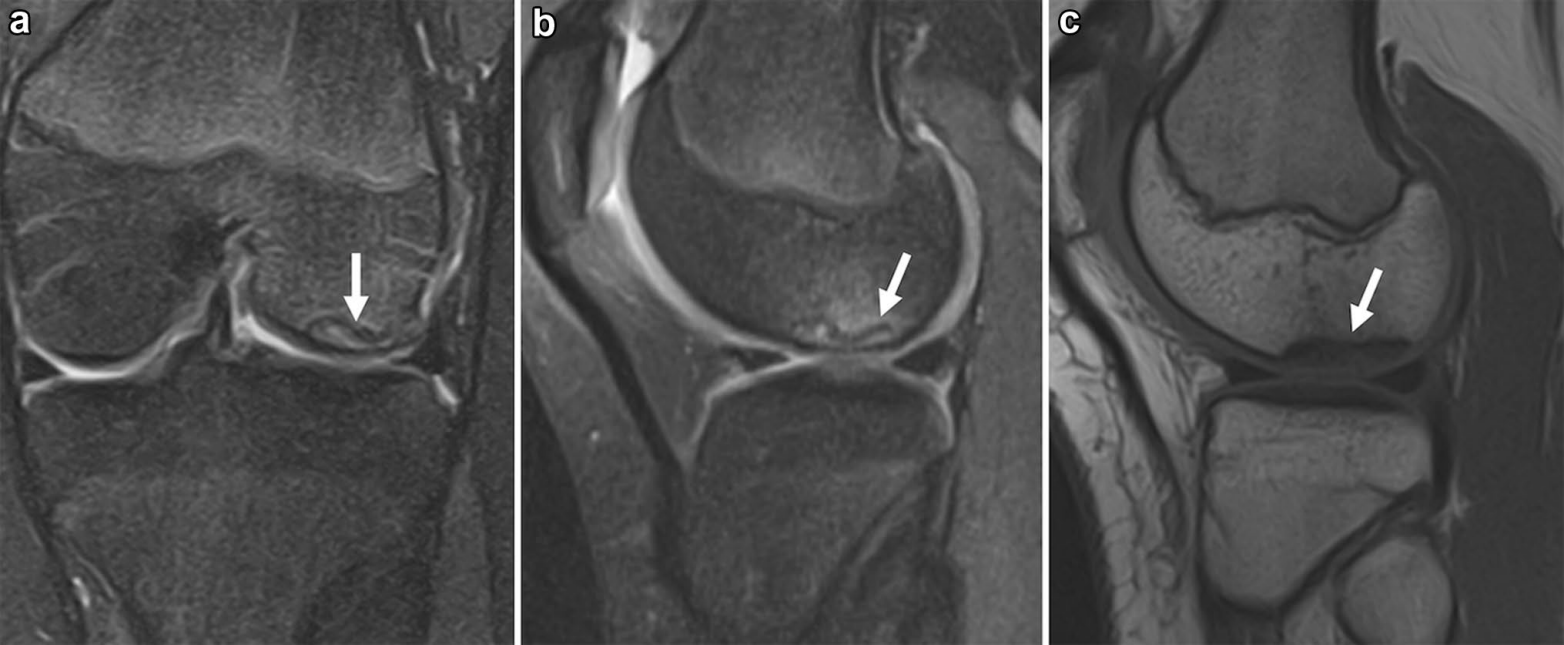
a Coronal FS-PDWI, b Sagittal FS-PDWI, and c T1-weighted image of a 14-year-old girl with stable OCD in the lateral femoral condyle in the left knee. The continuity of articular cartilage is preserved. There is no fluid signal or cyst formation around the lesion. Note the low signal band-like intensity observed between the lesion and the parent bone (arrows)
